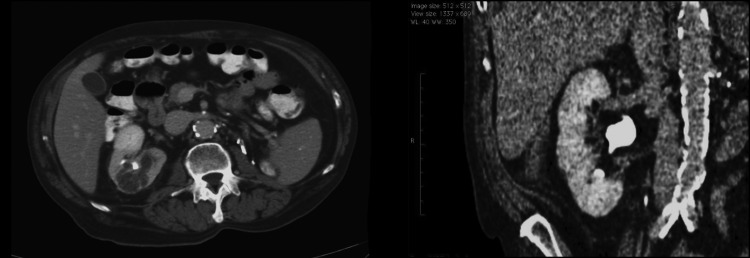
Post-treatment axial-contrasted abdominal CT scan demonstrating reduction in the right renal mass by 25%. Coronal view post-treatment of tyrosine kinase inhibitor. Regression of tumor away from the renal vein.
